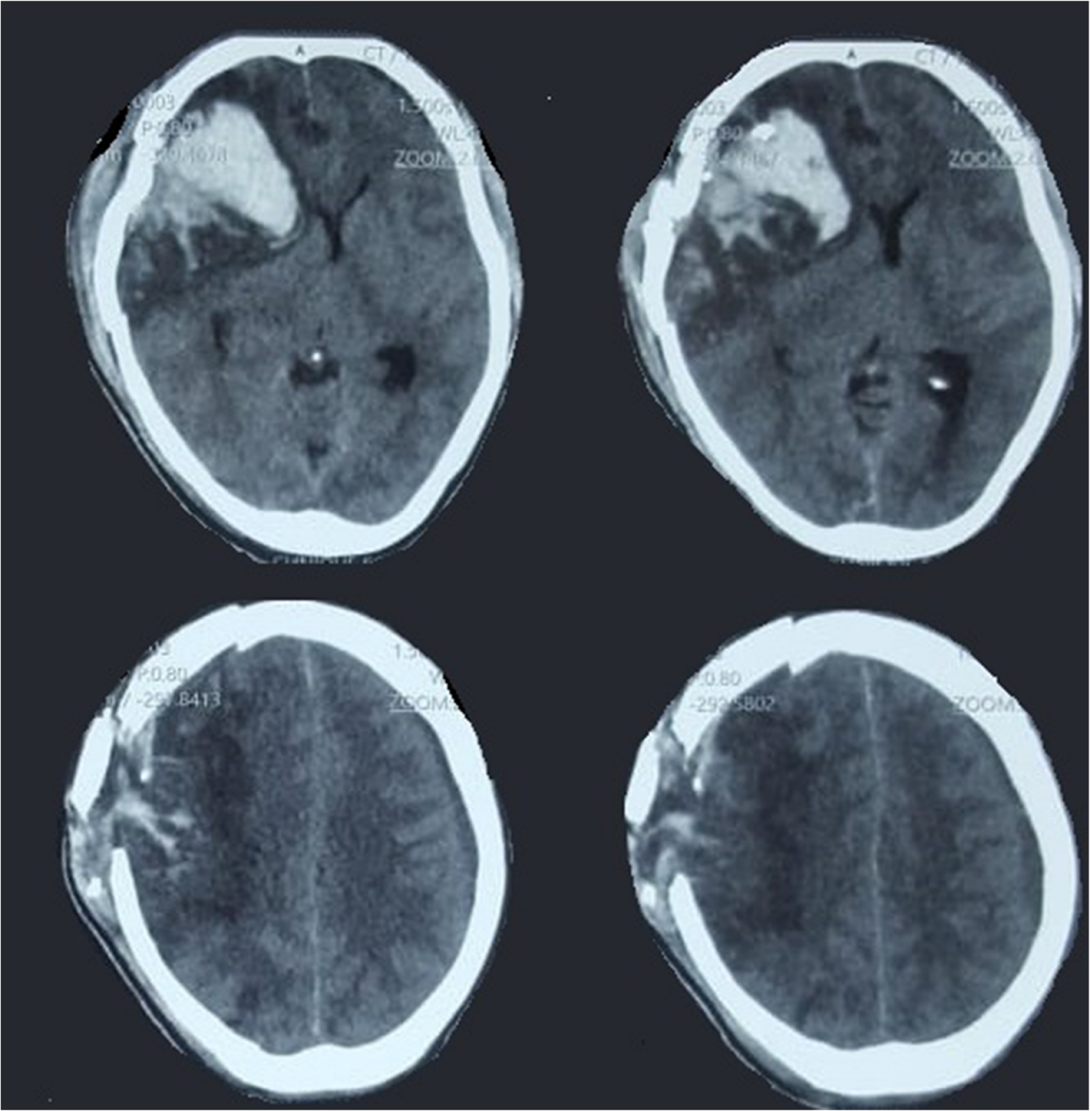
Brain Window CT of a patient with PCCI showing a comminuted right frontal fracture with an underlying frontal burst lobe with severe brain edema
